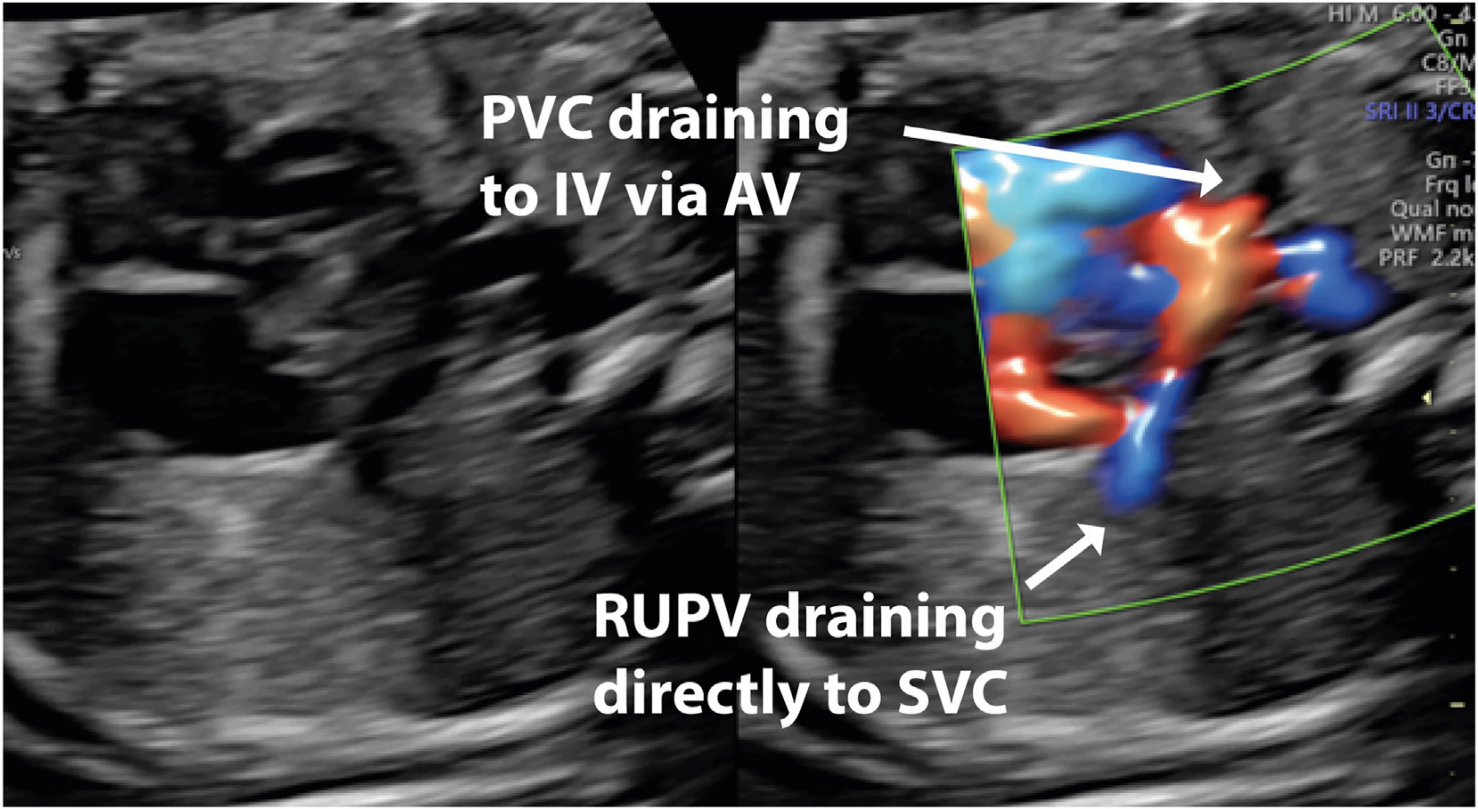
Two-dimensional fetal echocardiogram, obtained at 28 weeks of gestation, sagittal plane, without (*left*) and with (*right*) simultaneous color flow Doppler, demonstrates pulmonary veins joining the confluence, the ascending vein (AV) draining toward the IV, and the right upper pulmonary vein (RUPV) draining directly to the RSVC. *PVC*, Pulmonary venous connection; *SVC*, superior vena cava.
